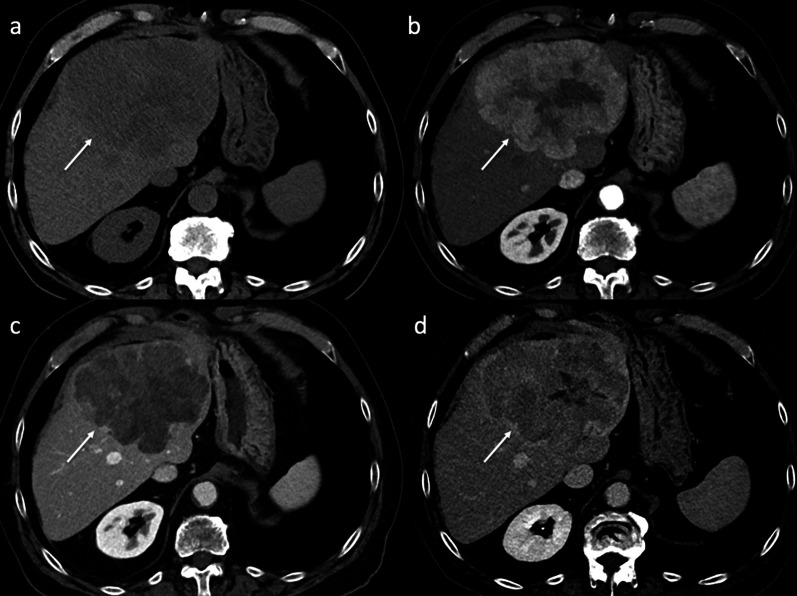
Liver recurrence. Unenhanced (a) and dynamic contrast-enhanced (b–d) axial CT imaging shows recurrent HCC (arrows) in both the hepatic lobes. The lesion shows the typical pattern of HCC: hypervascularity on the arterial phase (b), with washout in the portal venous (c) and 3-min late phases (d)
